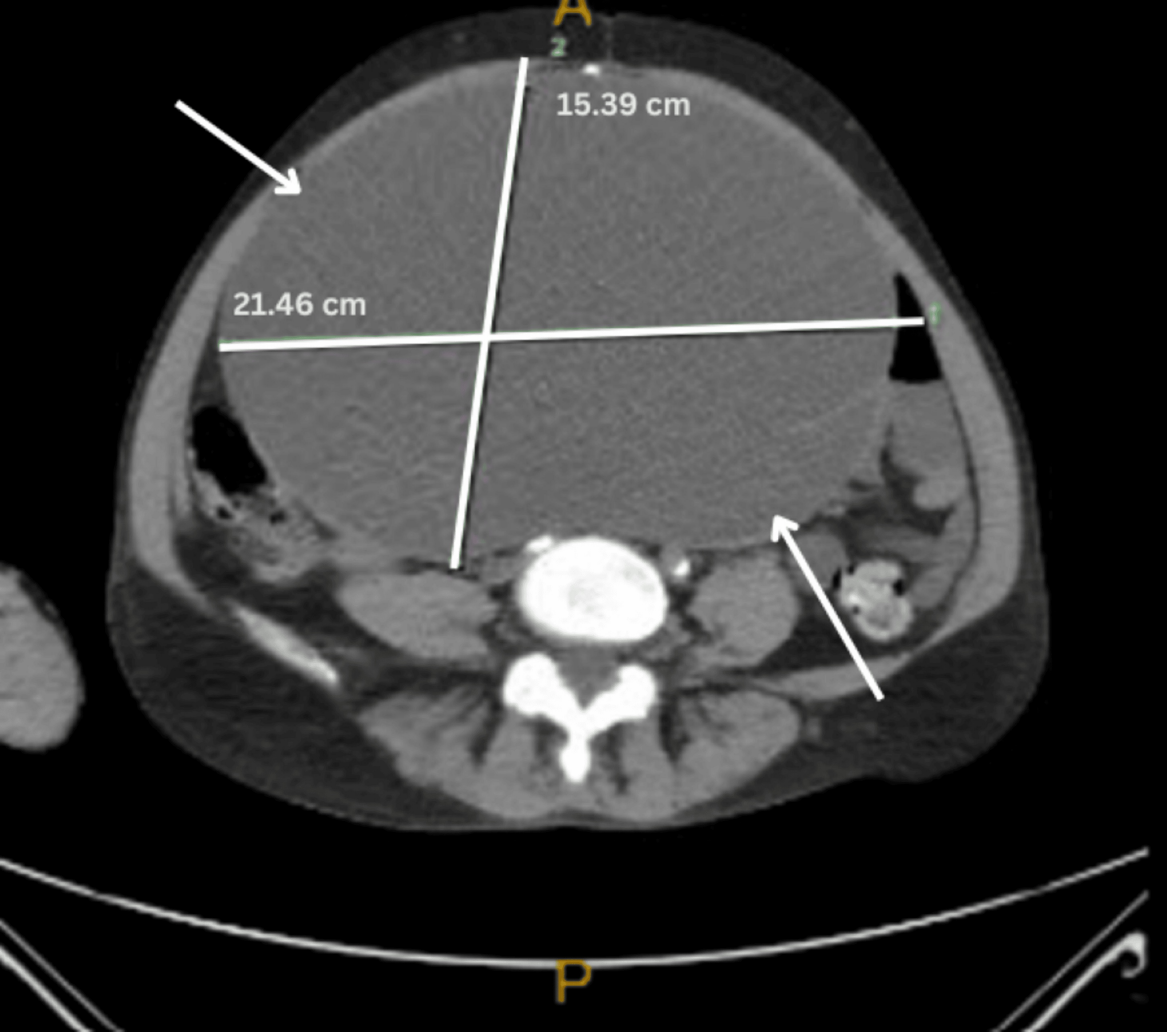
Transverse MRI image of the right borderline ovarian tumor measuring 21.46 cm x 15.39 cm.
